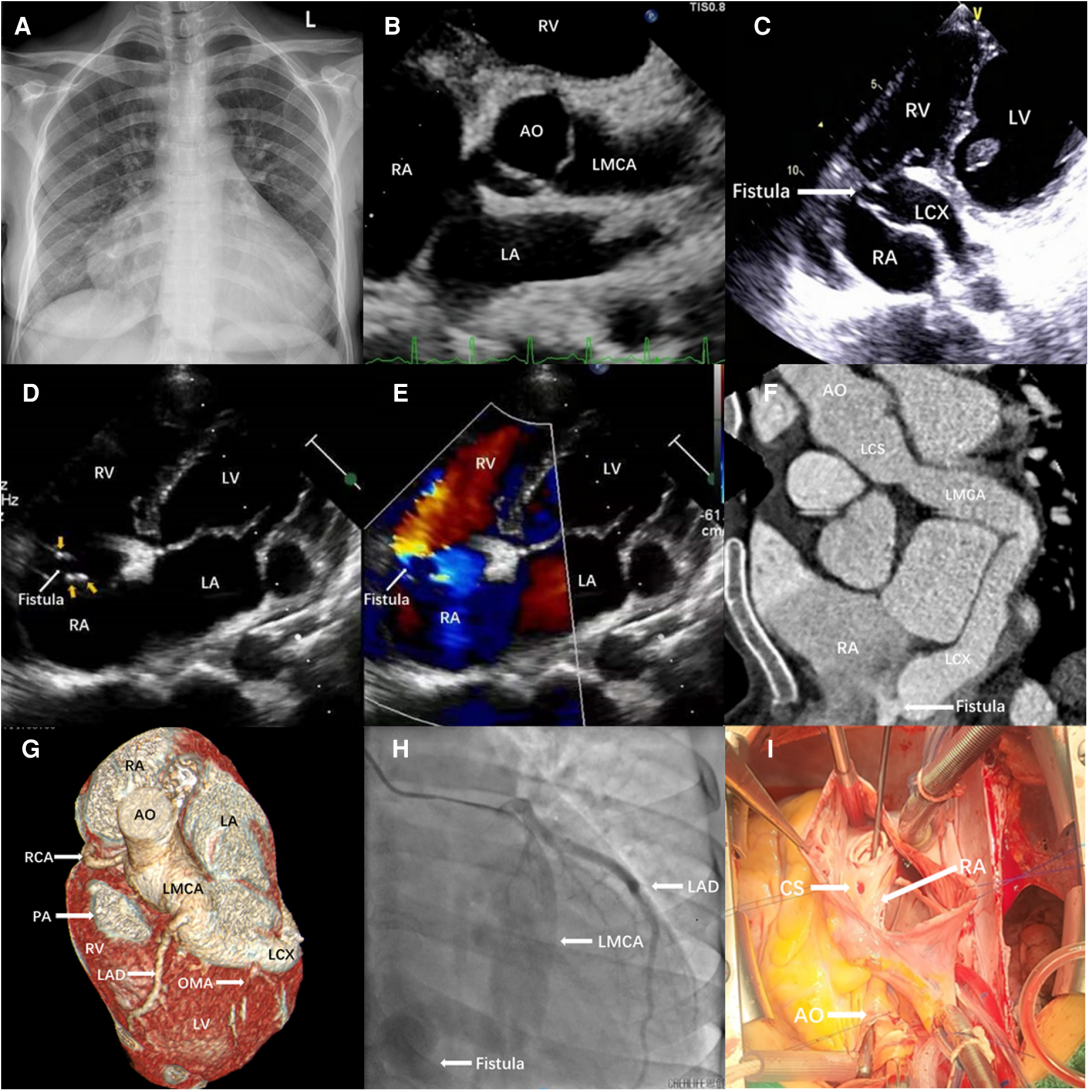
(A) Chest x-ray showed gross cardiomegaly. (B–E) Transthoracic echocardiography. (B) The parasternal short-axis image showed a large fistula, originating in LMCA. (C) Modified four-chamber view showed the fistula drained from LMCA into RA, adjacent to the coronary sinus. (D) A continuous systolic–diastolic flow inside the structure at color Doppler, which was confirmed at continuous wave Doppler directed from the LMCA toward RA. (E) Cardiac vegetations were found on the orifice of the fistula in the RA. Computed tomography angiography (F,G) and coronary angiography (H). A large fistula originating from LMCA and entering into RA was shown, and the LAD and OM arteries originate directly from LMCA (G,H). (I) Intraoperative findings. An abnormal vascular channel between left aortic sinus and RA (probe) was observed. AO, aorta; CS, coronary sinus; LA, left atrium; LAD, left anterior descending coronary artery; LMCA, left main coronary artery; LCX, left circumflex coronary artery; LV, left ventricle; OMA, oblique marginal artery; PA, pulmonary artery; RA, right atrium; RCA, right coronary artery; RV, right ventricle.
